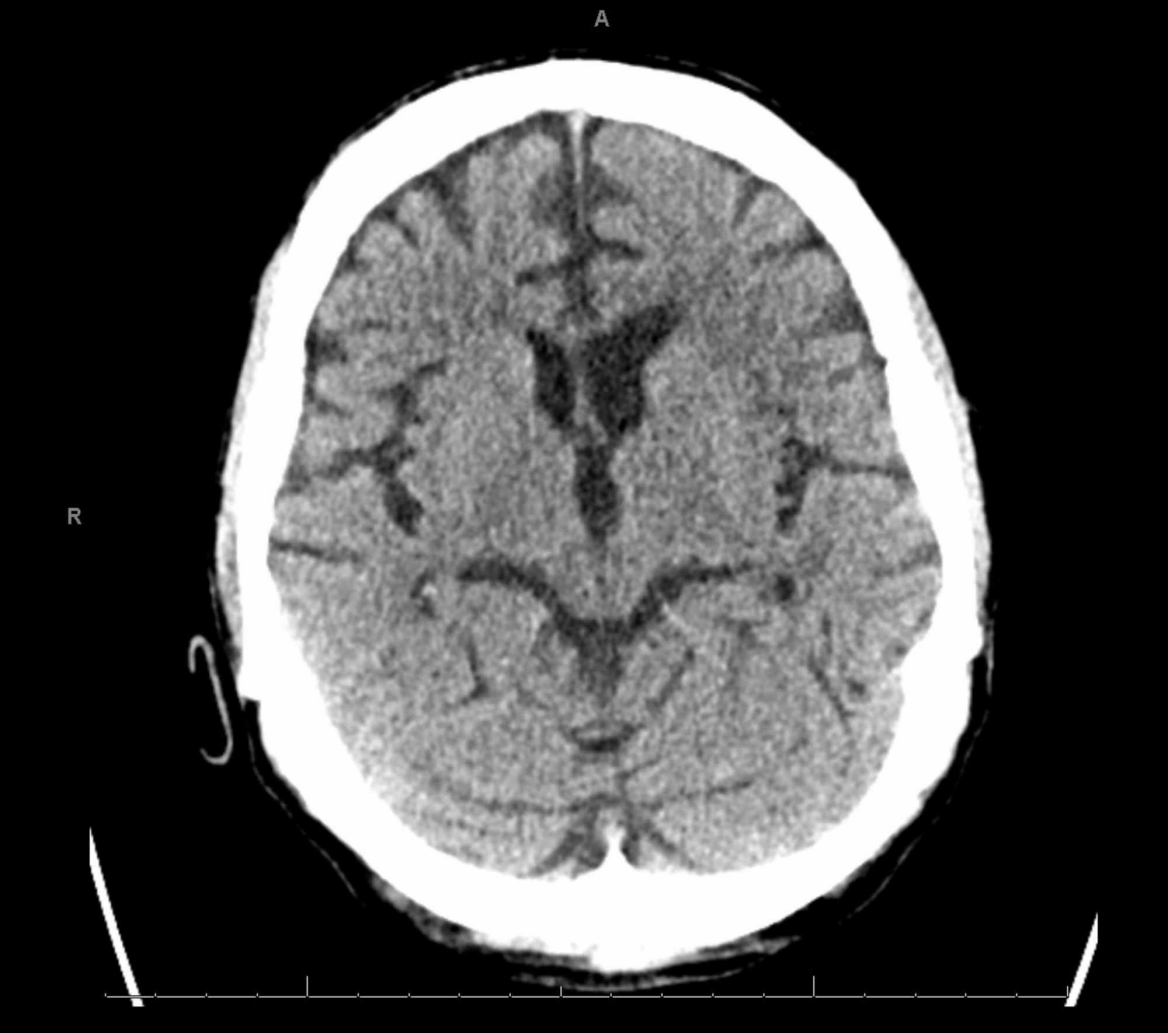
Computed tomography (CT) of the head without contrast on arrival
